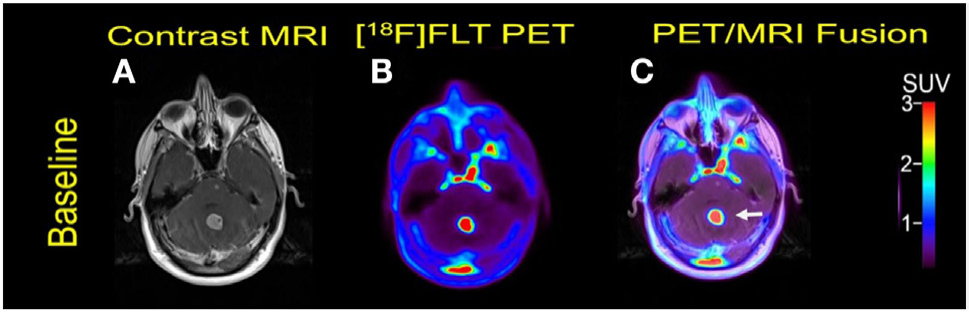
Case #4. Baseline magnetic resonance imaging (MRI) (A), fluorothymidine (FLT)–positron-emission tomography (PET) (B), and fused PET/MRI (C). The 1.6-cm cerebellar lesion (arrow) with FLT avidity showed SUV ratio of 18.2.
